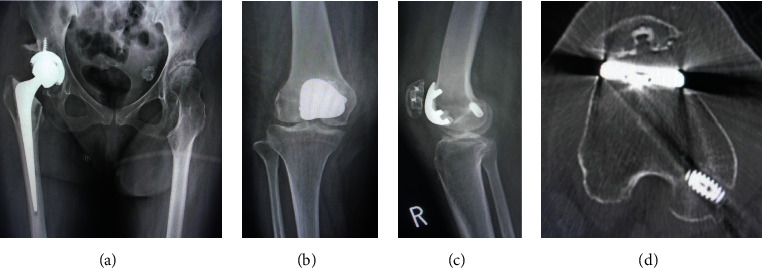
(a) X-ray of the hip after the first-stage surgery. (b) Postoperative X-ray of the right knee; anteroposterior view. (c) Postoperative X-ray of the right knee; lateral view. (d) Postoperative computed tomography of the right knee.
